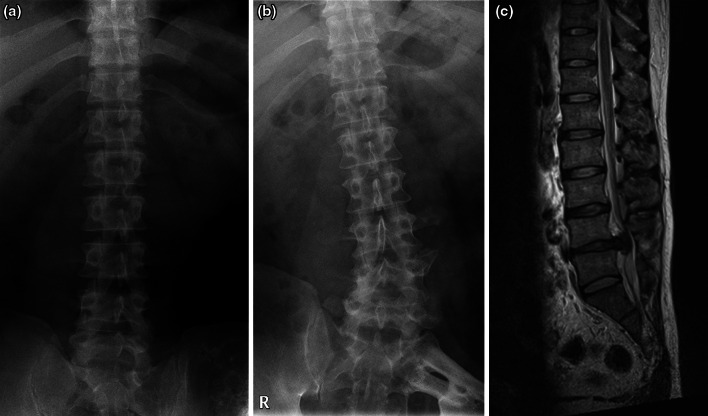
Plain radiograph at diagnosis of pelvic chondrosarcoma (a) and at diagnosis of lumbar disc hernia 6 years postoperatively (b). Gradual progression of scoliosis is evident. T2-weighted sagittal MR images demonstrate a severe lumbar disc hernia at the level of L4/5 (c)
